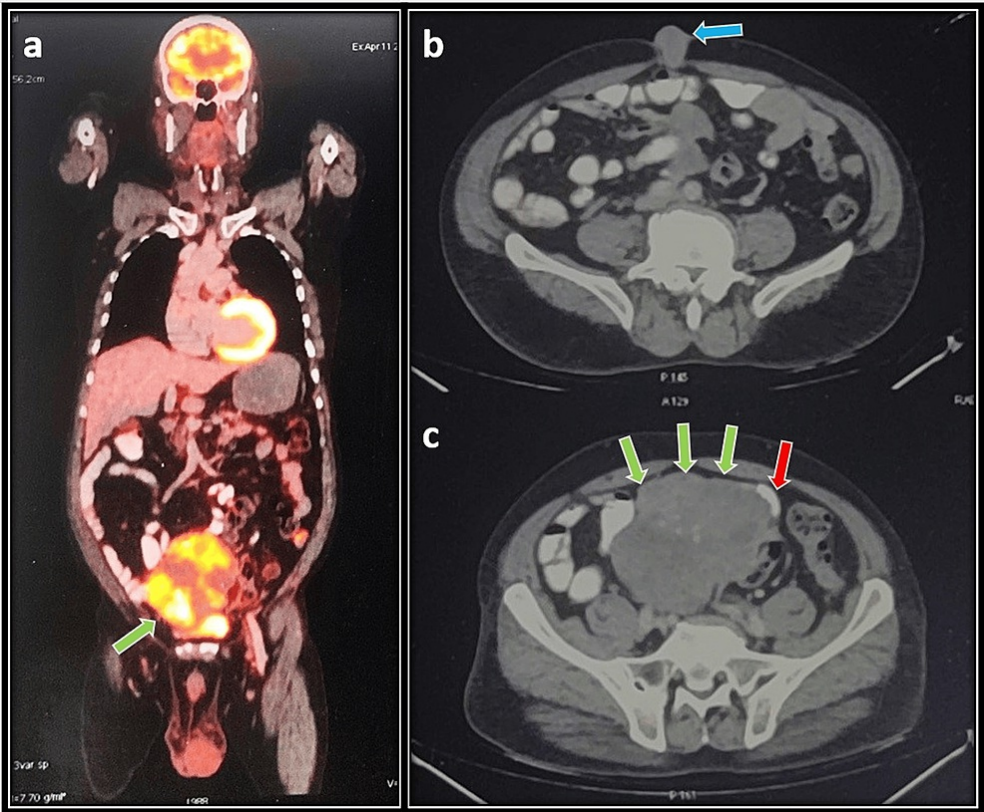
(Patient 1) a (green arrow): FDG-PET reconstructed image showing an enhancing mass lesion arising from the small bowel infiltrating the dome of the urinary bladder. b (blue arrow): Metastatic enhancing lesion at the umbilicus presenting as an umbilical nodule. c (green arrows): GIST arising from small bowel loops (red arrow). FDG-PET: fluorodeoxyglucose positron emission tomography, GIST: gastrointestinal stromal tumor
